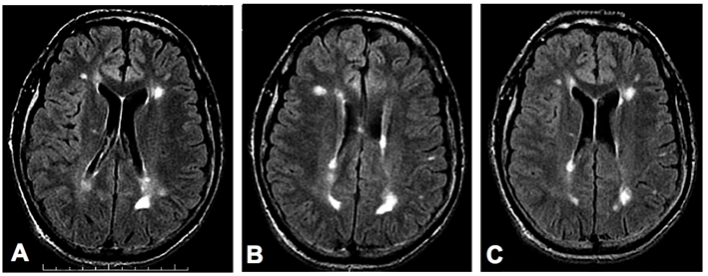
MRI Images obtained before (Panels A and B), and seven months after (Panel C) the stem cell treatment of patient 2. Panels A and B: Consecutive axial FLuid-Attenuated Inversion Recovery (FLAIR) images through the lateral ventricles show multiple small patches of bright signal in the periventricular and subcortical white matter, consistent with plaques of multiple sclerosis. Panel C: Axial FLAIR image shows no significant change in the multiple periventricular and subcortical white-matter plaques. (For the comparison, note that this slice is positioned similar to slice A but at slightly different scanning-angle, so it includes lesions of both slices A and B.).
